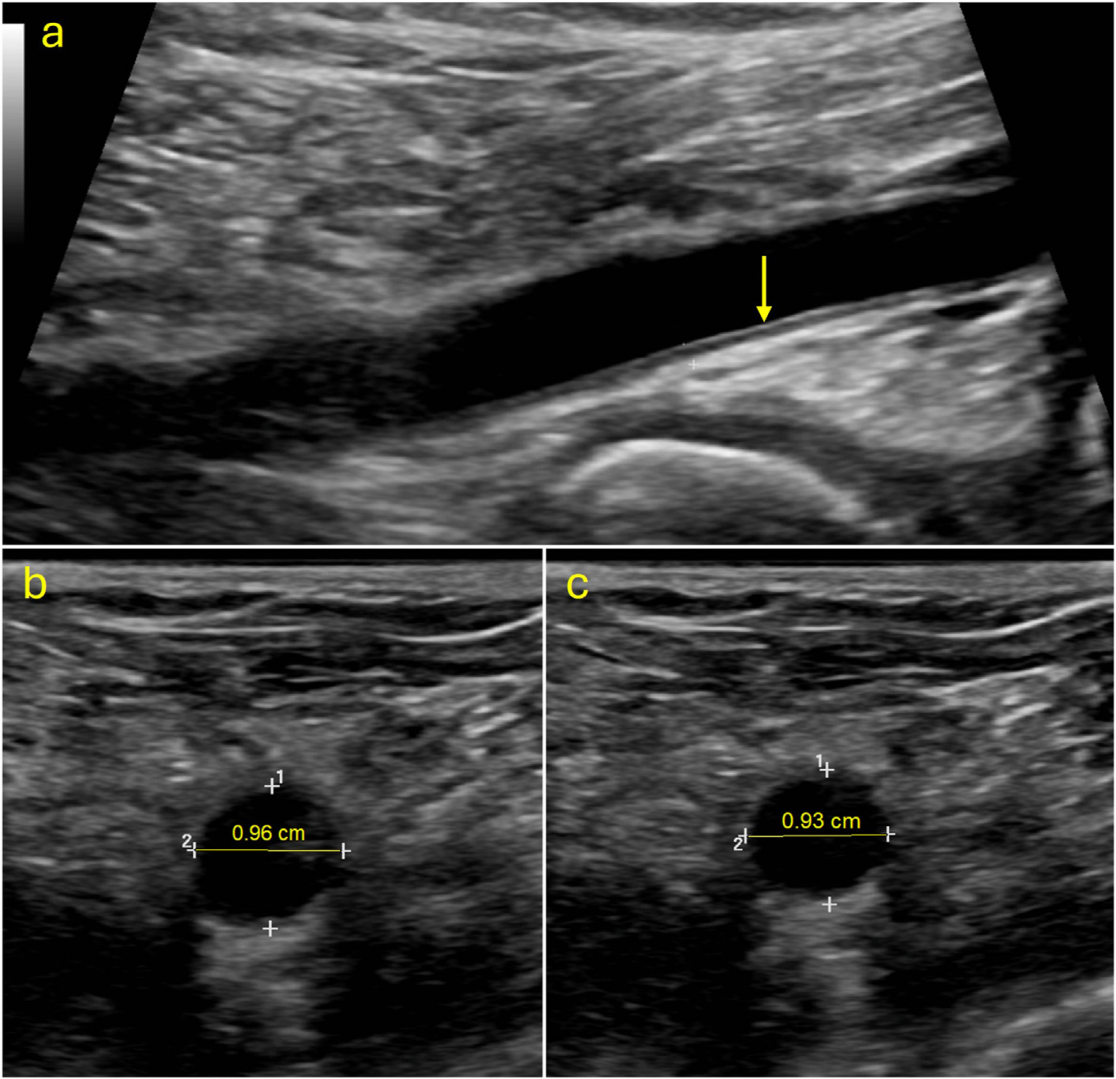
Sonographic images of structural vessel properties captured using the GE Logiq9 system. (a) Intima-media thickness of the popliteal artery (arrow). (b) Systolic cross-section of the common femoral. (c) Diastolic cross-section of the CFA. Area change was measured using the mean cross-sectional diameter differences between systole and diastole.
